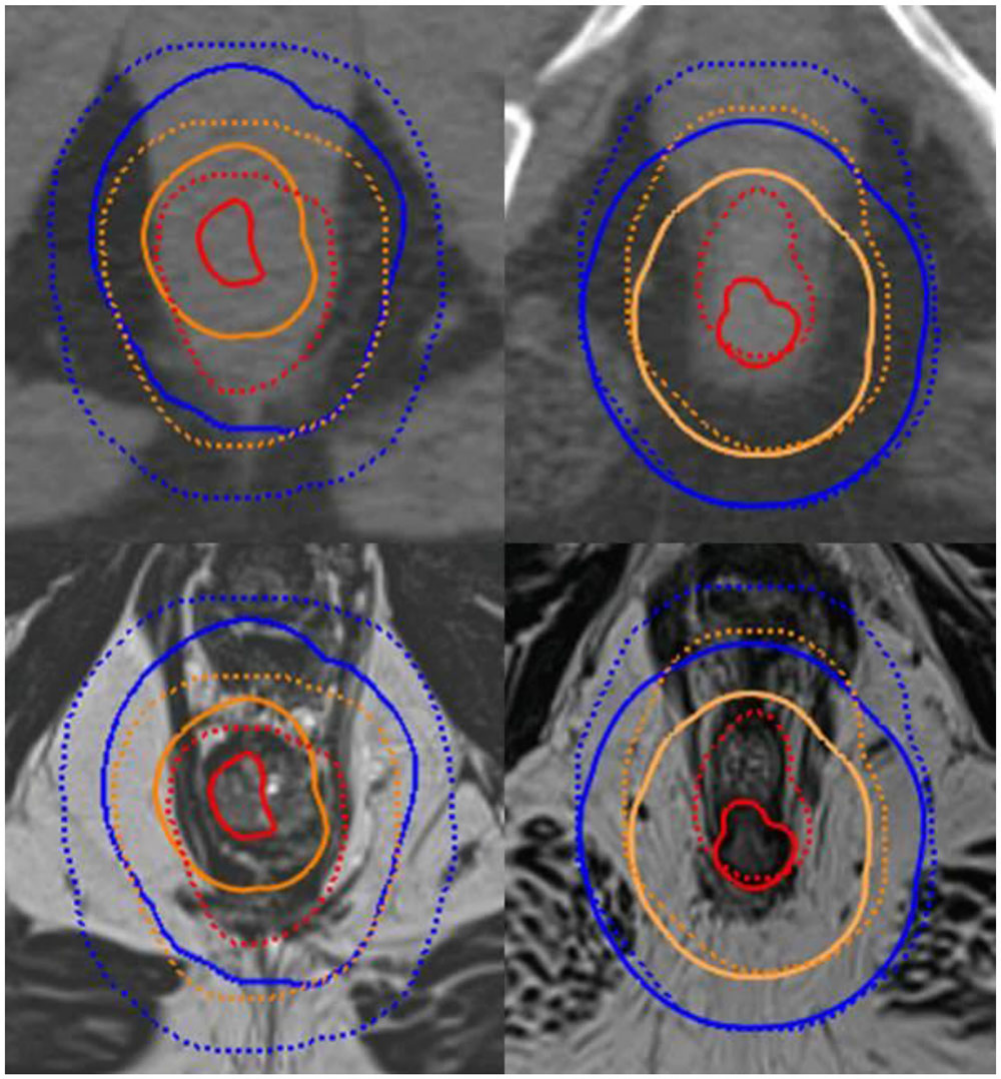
Comparison of anus (right) and rectum (left) cancer GTVs (red), CTVAs (orange), and primary PTVs (blue) for MR (bold) versus CT (dotted) delineations on CT (top) and T2 SPACE MR (bottom) datasets
